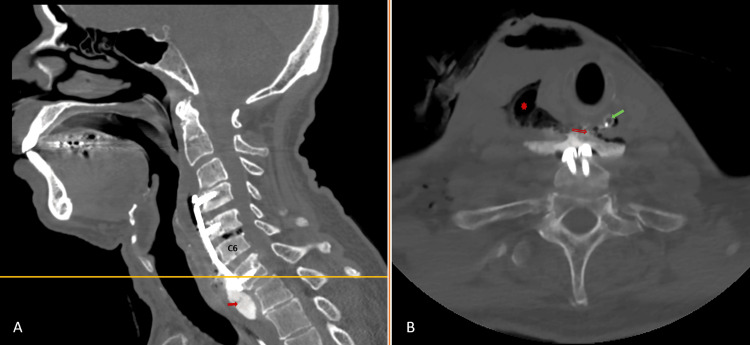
Neck CT with oral Gastrografin taken when the output of the surgical drain was increasing following the first incision and drainage procedure. (A) Sagittal view showing the contrast leak (red arrow). (B) Axial view taken at the level of the seventh cervical vertebra (the yellow line in (A)), where the site of esophageal injury/leak is present (red arrow). The green arrow is pointing at the nasogastric tube.
